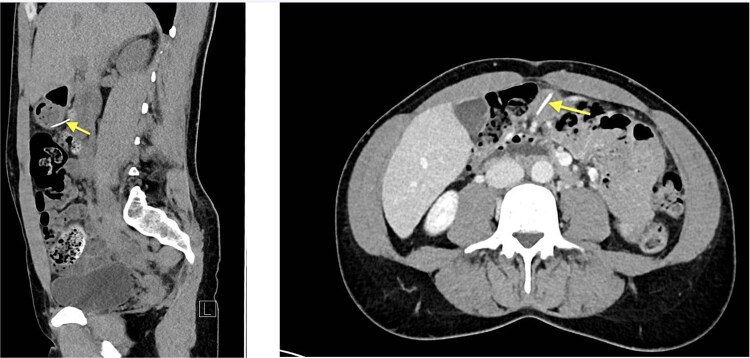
Abdominal CT (03.05.2024), hyperdense foreign body (metallic wire).
